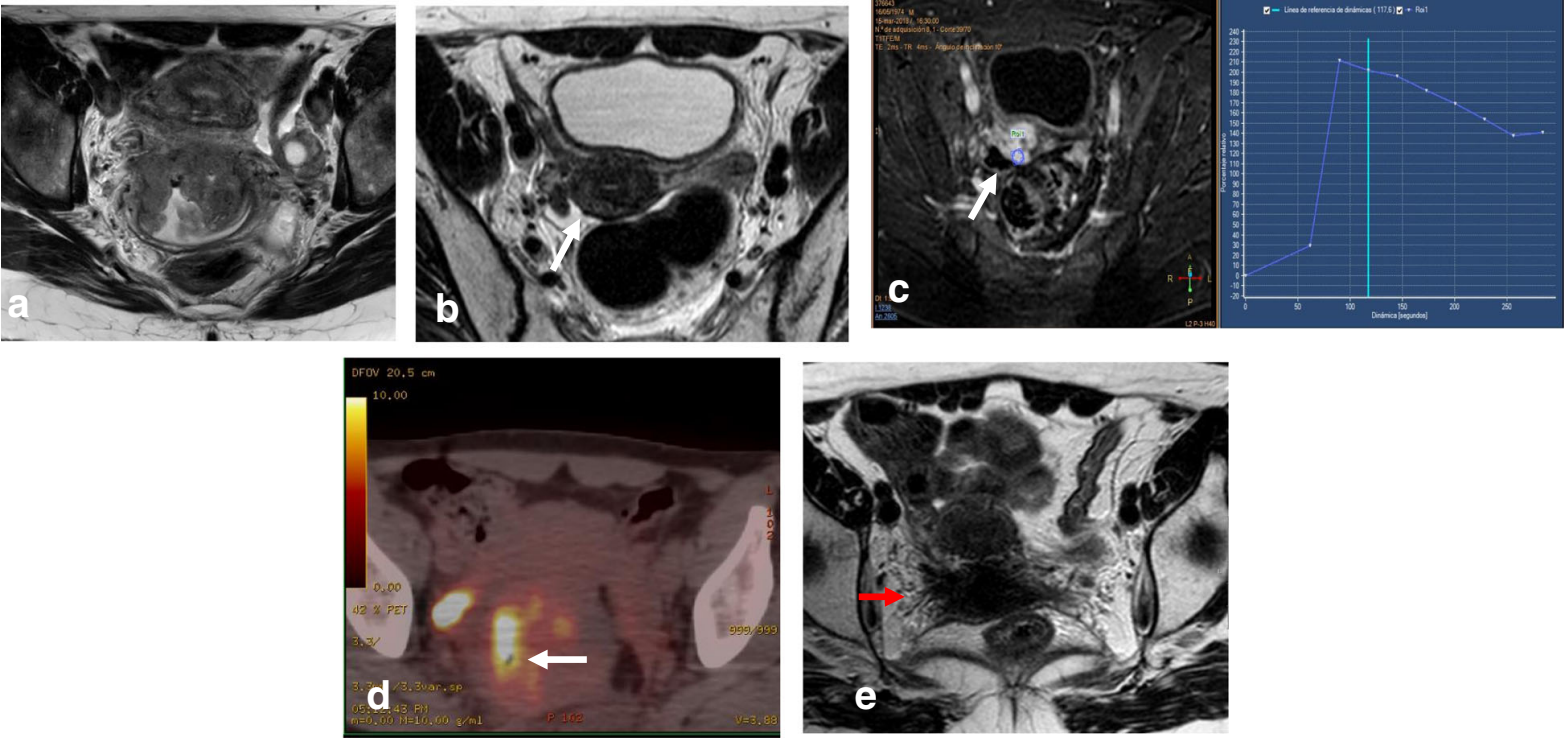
Cervical cancer treated with chemotherapy and radiotherapy. a Axial oblique T2WI at diagnosis shows a cervical mass. b, c, d A post-treatment residual mass (arrows) is detected on the right posterior aspect of the cervix by axial oblique T2WI (b), DCE and a type 3 time-intensity curve (c) and PET/CT (d). e Axial oblique T2WI at 6 months after treatment establishes cervical and right parametrium fibrosis (red arrow) and shows no residual mass
